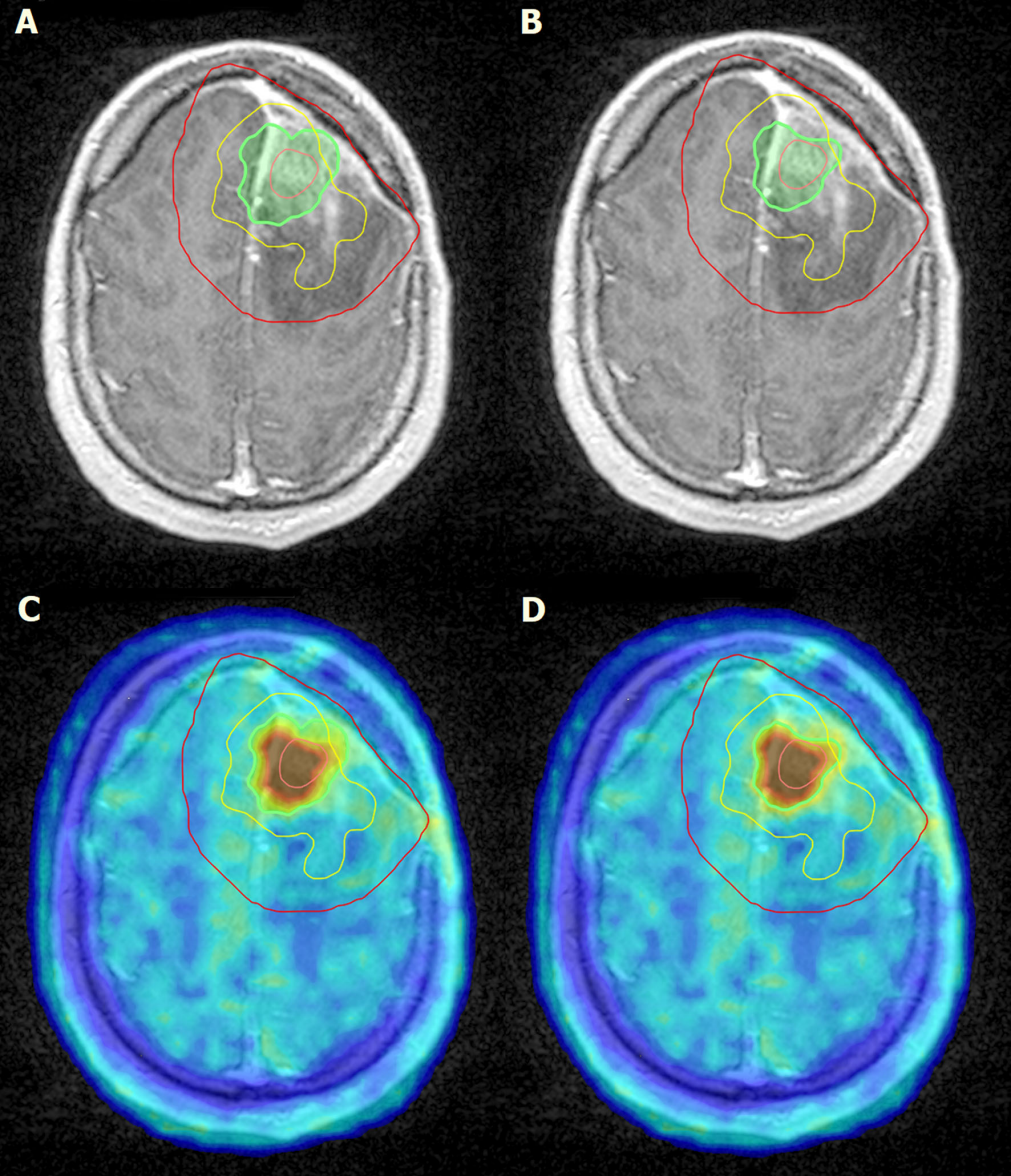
Left frontal post craniotomy status. Inhomogeneous, mainly centrally, moderate enhancement of contrast material is observed on T1-weighted post contrast MRI images. The lesion in the left hemisphere is surrounded by edema (A, B). Irregularly shaped intense, focal 18F-FDOPA accumulation can be detected on the left side of the brain frontally, above the level of lateral ventricles (C, D). Pink line, GTV; green line, BTV 1.7 (A, C); green line, BTV 2.0 (B, D); red line, PTV; yellow line, recurrence.
